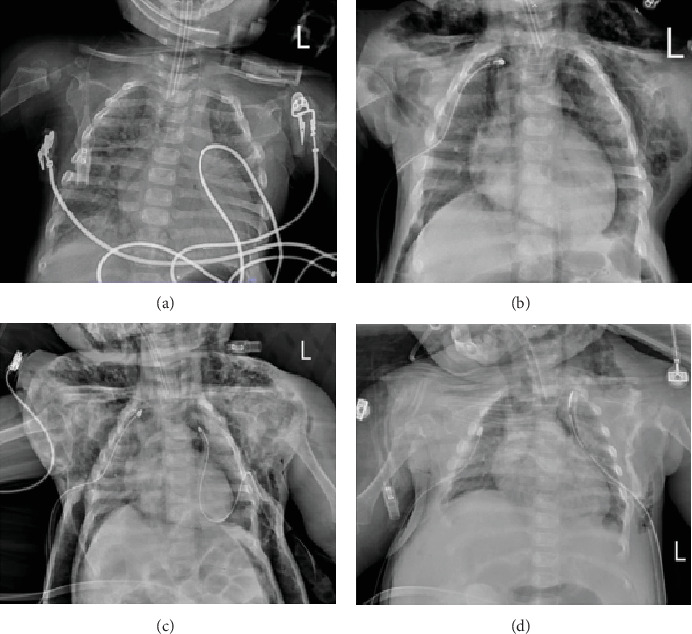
(a) Chest x-ray following intubation at the emergency department. (b) Chest x-ray after to the recognition of subcutaneous emphysema and the insertion of intercostal drainage in the right chest wall. (c) Chest x-ray showing the progression of subcutaneous emphysema during conventional mechanical ventilation mode. (d) Chest x-ray after the use of high-frequency oscillatory ventilation and the insertion of a butterfly needle into the subcutaneous area for air drainage.
